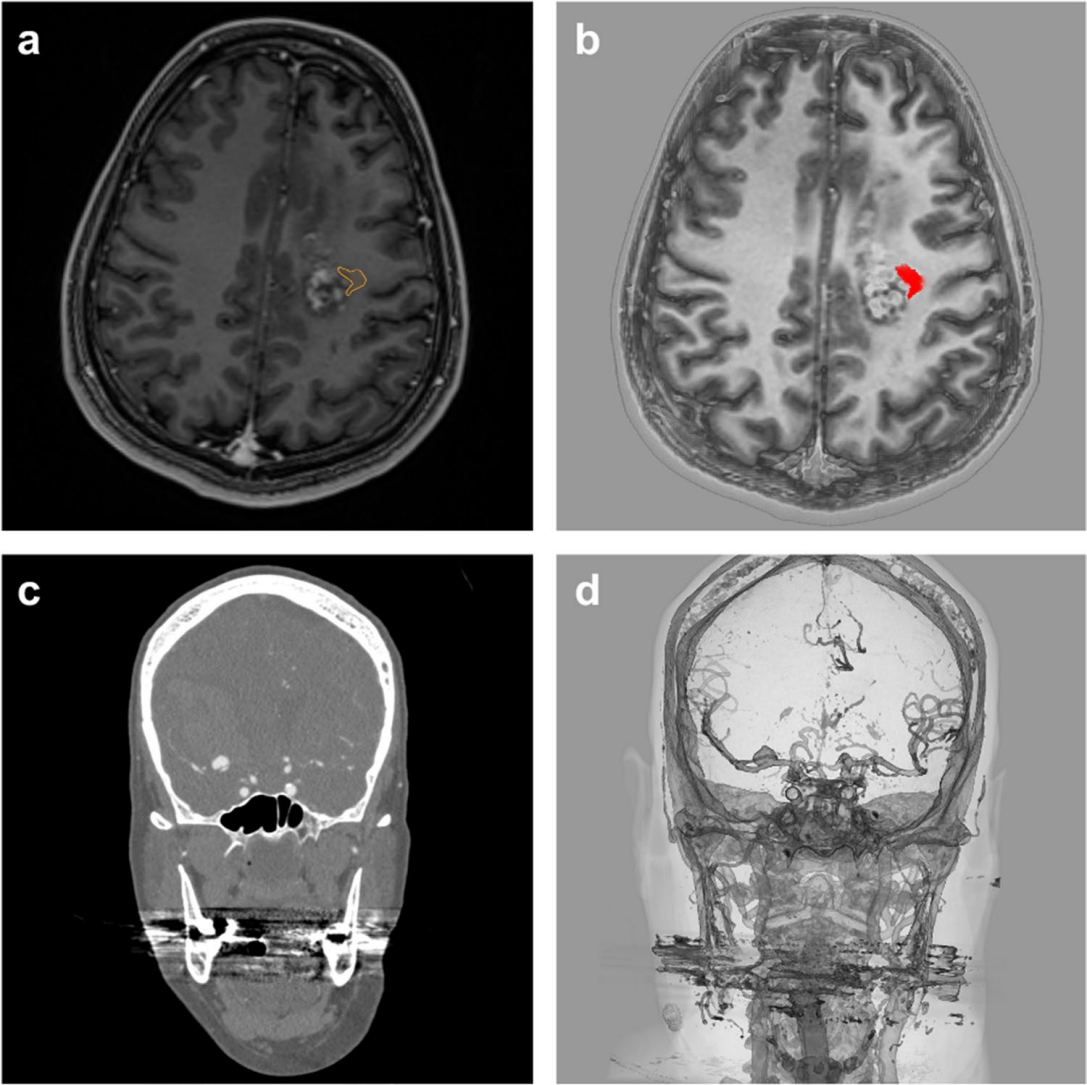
In the top row (a + b), an axial view of the T1-weighted magnetic resonance imaging of the central tumor (tumor 1), monoscopically with Brainlab (a) and stereoscopically with VP Reveal (b). The tract is shown in orange for Brainlab and red for VP Reveal. In the lower row (c + d), a coronal view of the computed tomography angiography of a middle cerebral artery bifurcation aneurysm (aneurysm 2), monoscopically with Brainlab (c) and stereoscopically with VP Reveal (d)
